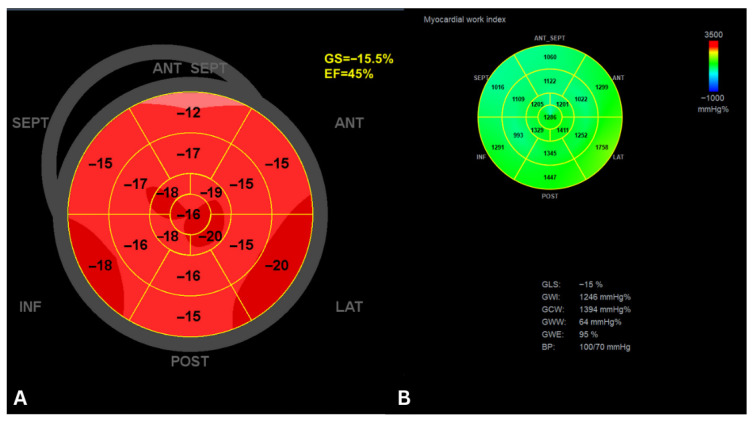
Myocardial work analysis in a patient with heart failure and mildly reduced EF. (A) Bullseye plot from speckle-tracking echocardiography showing a GLS of −15.5%, indicating moderately reduced systolic function. (B) Myocardial work analysis plot indicates largely preserved contractile efficiency with minimal wasted work. GWI has a value of 1246 mmHg%, indicating a reduced risk of hospitalization for heart failure. GS, global longitudinal strain; ANT, anterior; LAT, lateral; POST, posterior; ANT SEPT, antero-septal, INF, inferior; SEPT, septal.
